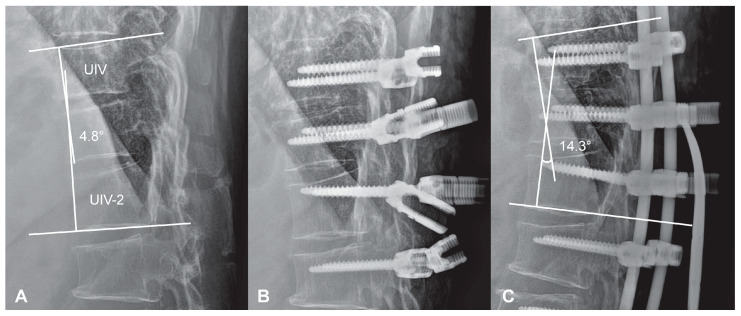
Intraoperative application of the TLJ distraction technique. (A) Preoperative prone lateral radiograph showing the baseline kyphotic angle between UIV and UIV–2 (4.8°). (B) Pedicle screws inserted at UIV, UIV–1, and UIV–2 with sagittal orientation tailored to accommodate a pre-contoured rod replicating mild kyphosis. Screw heads are aligned to facilitate gradual distraction without anterior over-angulation. (C) Final construct demonstrating controlled kyphotic correction (14.3°) achieved via distraction between UIV–UIV–1 and UIV–1–UIV–2 under fluoroscopic guidance and sequential tightening.
